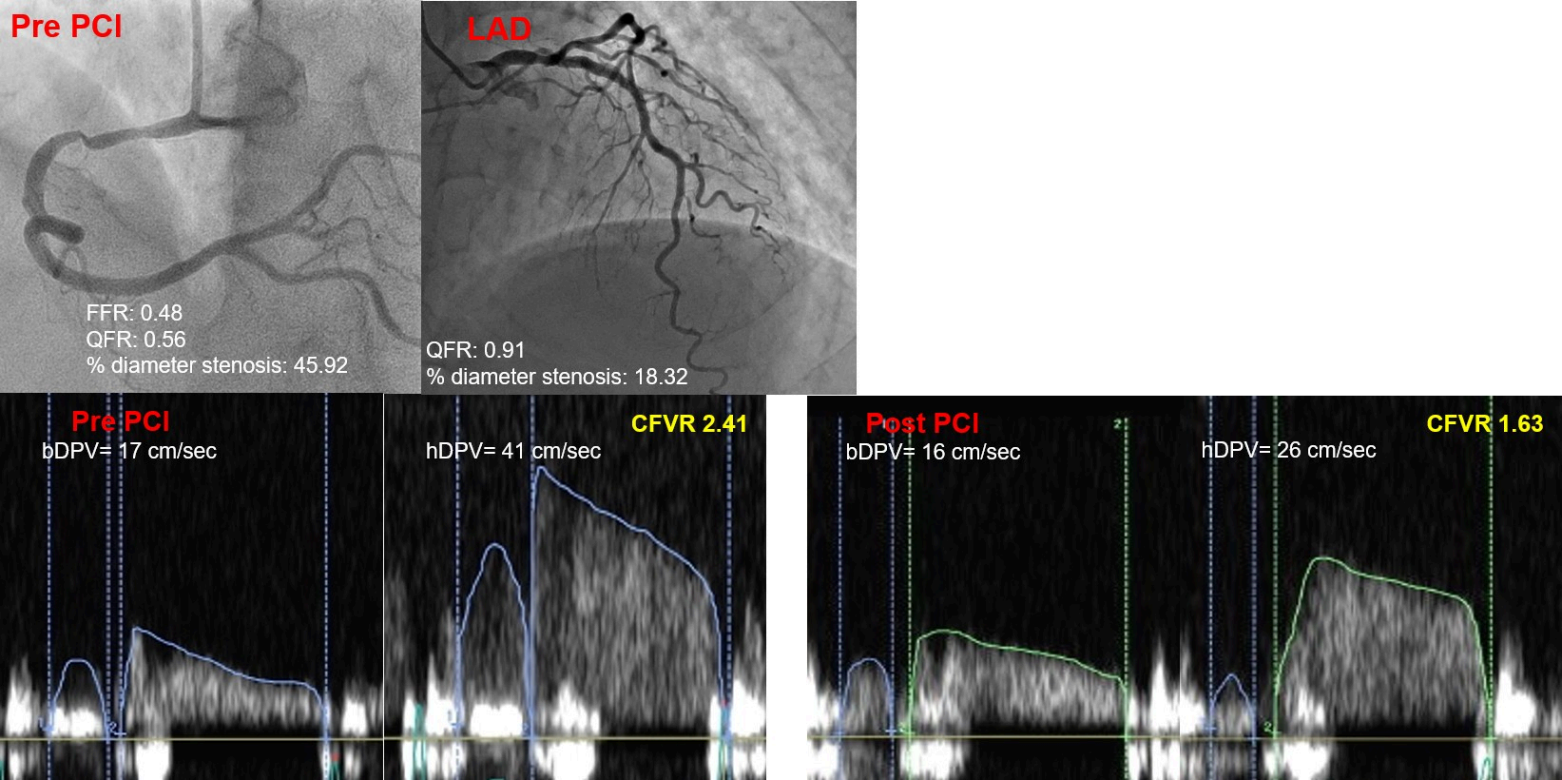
A representative case of a change in LAD-CFVR after non-LAD PCI by transthoracic Doppler echocardiography. An 80-year-old man without a significant stenosis in LAD. After successful RCA-PCI, LAD-CFVR decreased from 2.41 to 1.63 due to the reduction of hDPV. LAD, left anterior descending artery; CFVR, coronary flow velocity reserve; PCI, percutaneous coronary intervention; RCA, right coronary artery; hDPV, hyperemic peak diastolic velocity.
